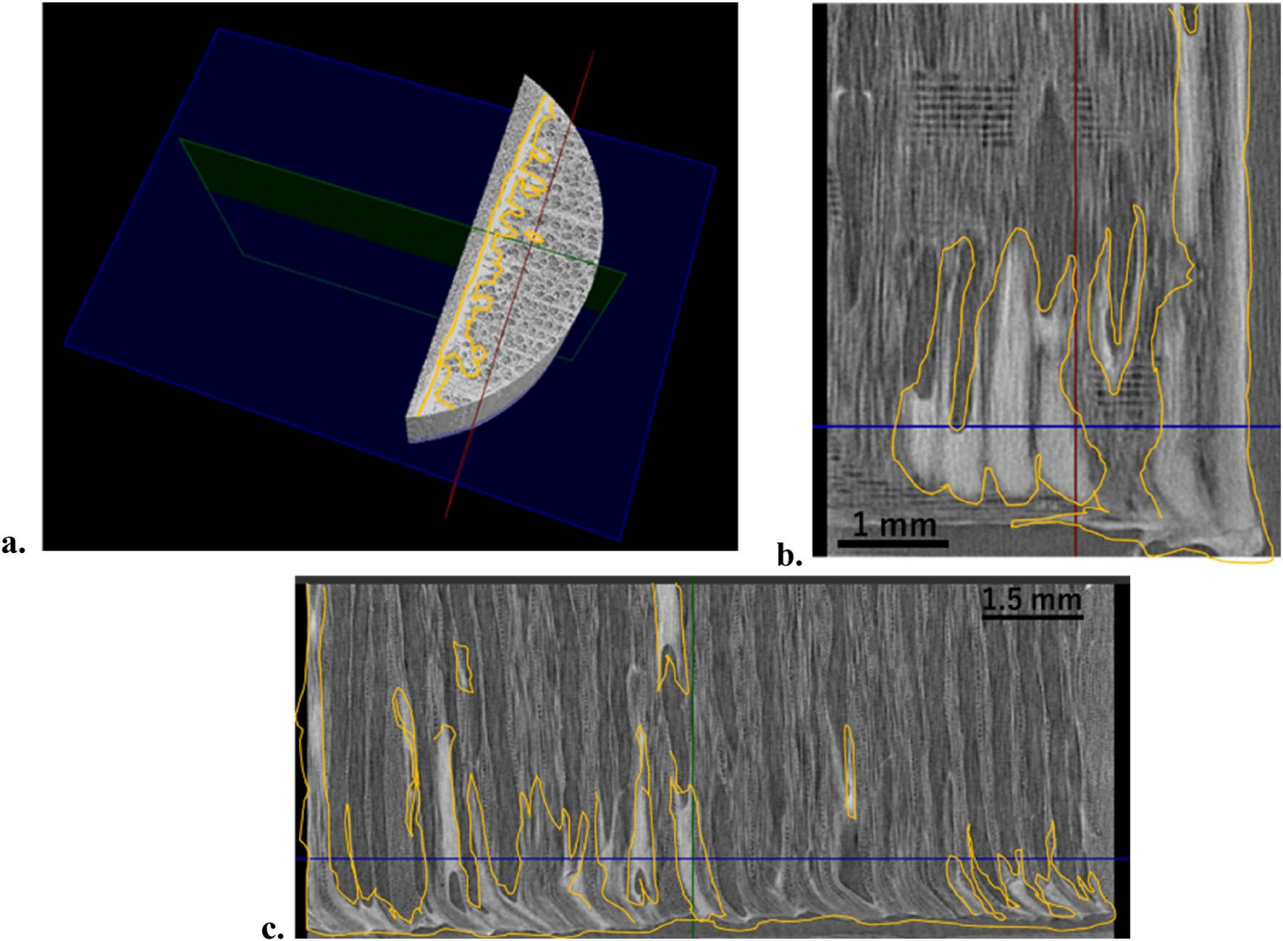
3D X-ray observation of coating penetration. (a) 3D image stack of veneer with white coating, (b) 2D representative image of a radial section were obtained from the green cut section of the 3D image, (c) 2D representative image of a tangential section were obtained from the red cut section of the 3D image. The yellow line annotated the coating part.
